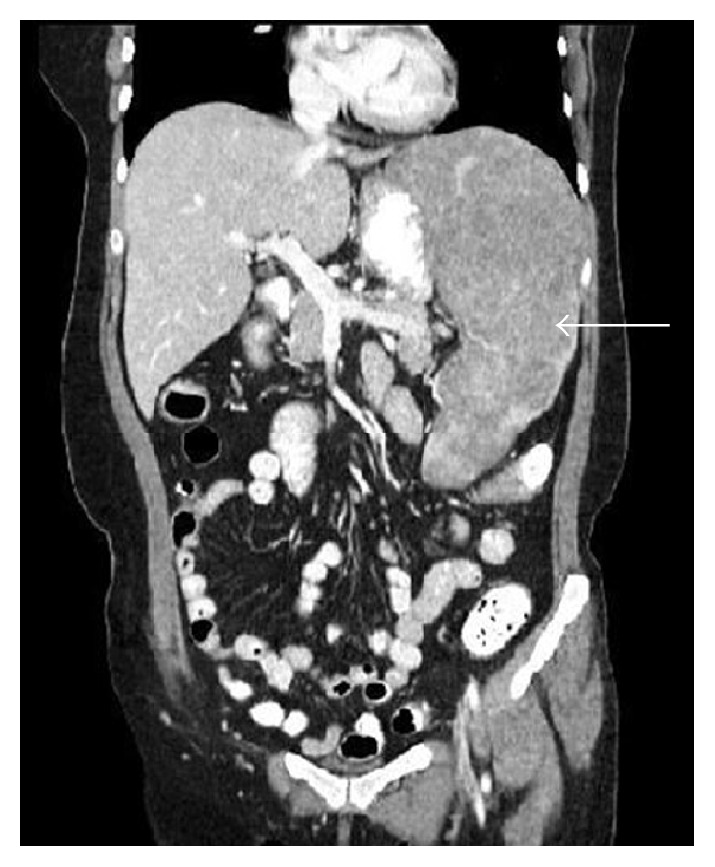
CT scan shows marked enlargement of the spleen and heterogeneous composition and demonstrates low attenuation masses.
